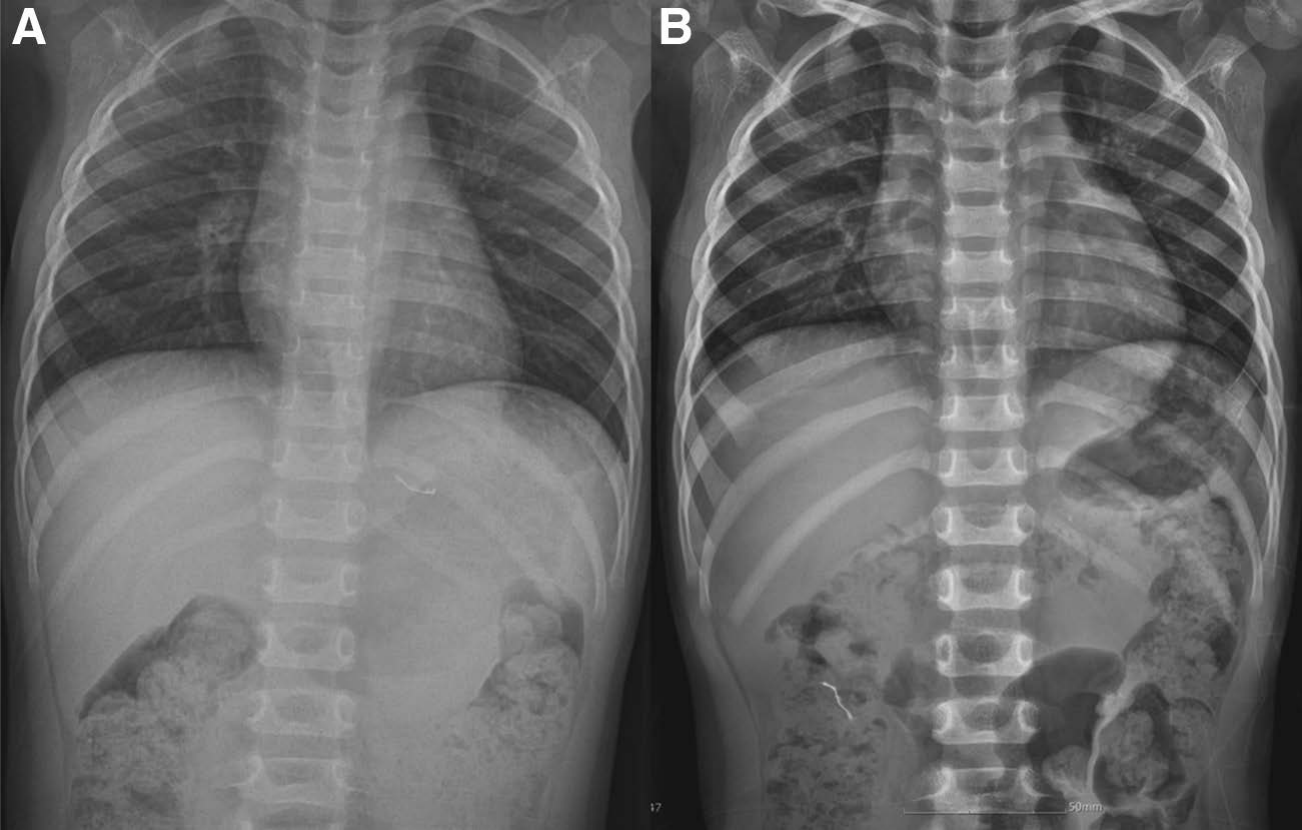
(A) Chest radiographs in the ED revealed a swallowed orthodontic splint in the stomach. (B) A follow-up radiograph obtained 11 h later showed the orthodontic splint in the ascending colon without any signs of intestinal perforation or associated complications. ED = emergency department.
